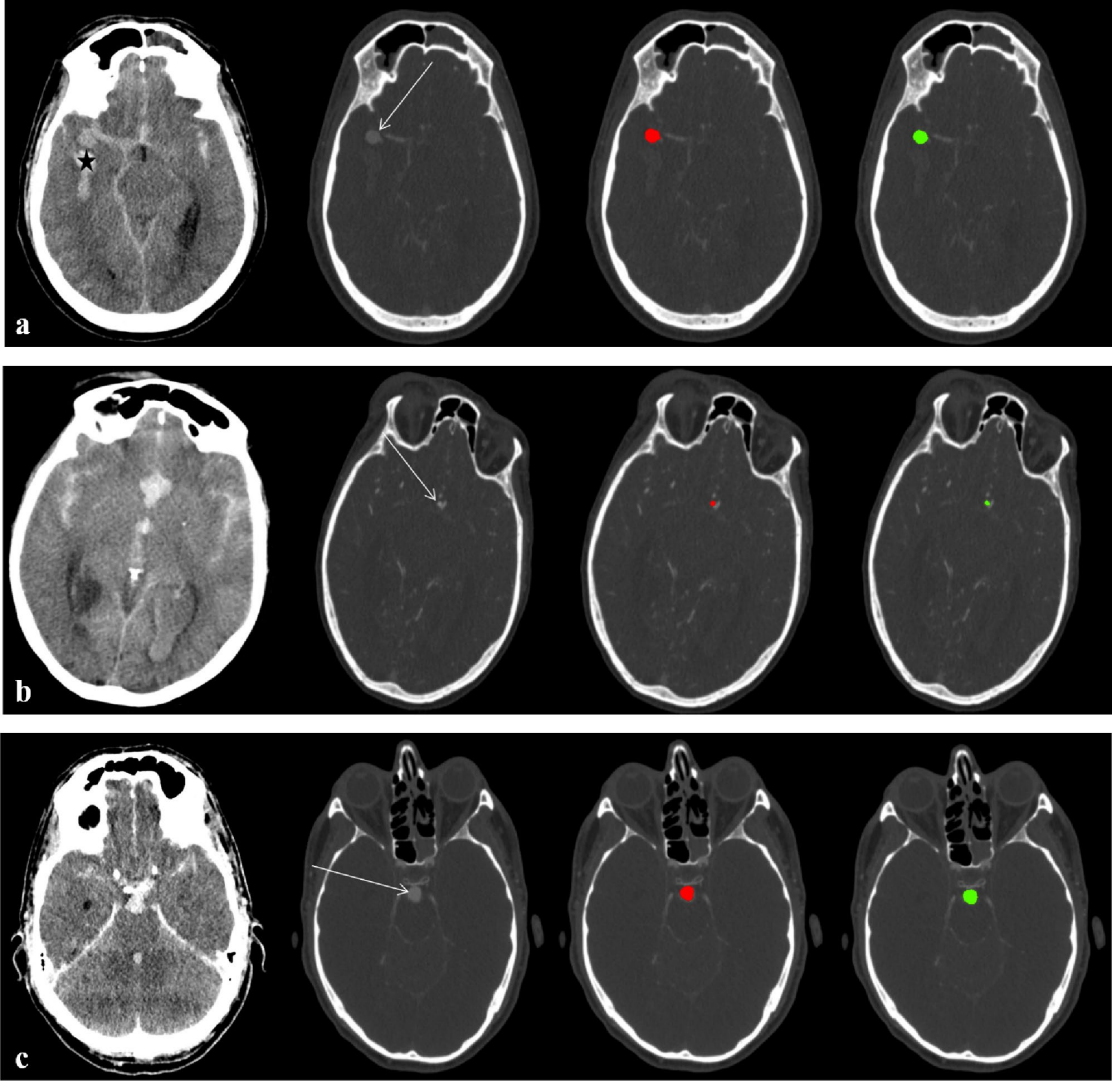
(a) Fifty-one-year-old male with Fisher 4 bleeding (* = intraventricular bleeding on axial unenhanced CT) and an aneurysm of the right middle cerebral artery (arrow) on axial CTA source images. The DLM-Ens (green) detects and segments the aneurysm (volume based on manual segmentation: 424.7 mm^3^) with high overlap (DSC of 0.94) compared to manual segmentations (red). (b) Sixty-three-year-old female with aSAH on axial unenhanced CT and an anterior communicating artery aneurysm (arrow) on axial CTA source images. Albeit being of small size (volume based on manual segmentation: 25.5 mm^3^), the DLM-Ens (green) detects and segments (DSC of 0.72) the aneurysm with high overlap compared to manual segmentations (red). (c) Fifty-one-year-old male with aSAH on axial unenhanced CT and a large basilar tip aneurysm (arrow; volume based on manual segmentation 419.6 mm^3^) on axial CTA source images. Compared to manual segmentations (red), the DLM-Ens (green) provides accurate detection and segmentation (DSC of 0.90).
